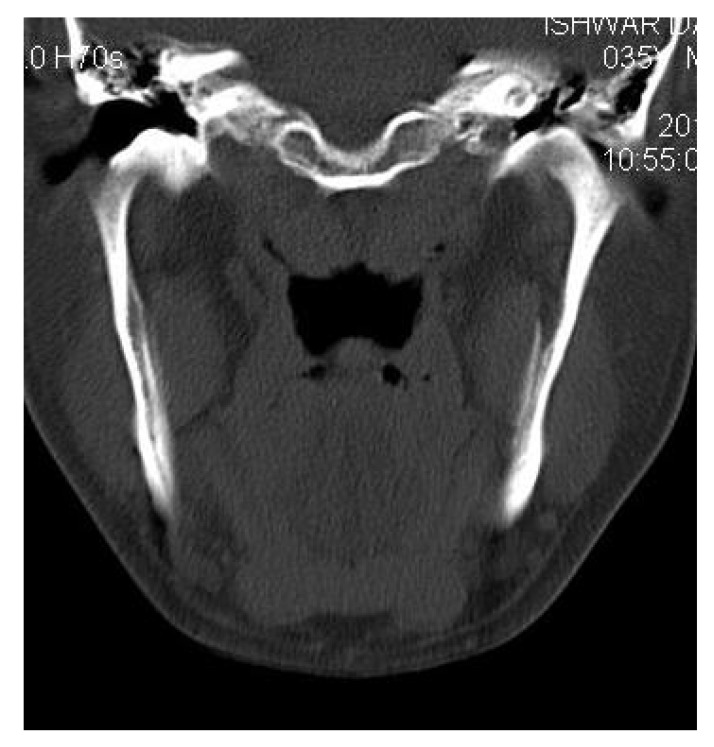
Coronal CT section revealing the normal TMJ.
